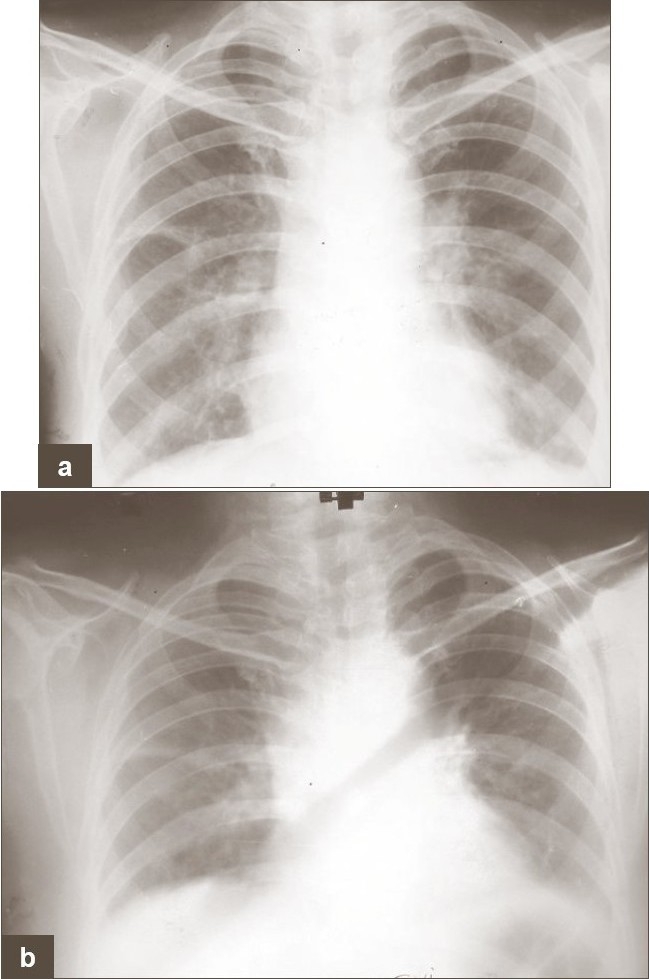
(a, b) Follow-up chest X-ray revealed resolution of pleural effusion as well as parenchymal shadows
